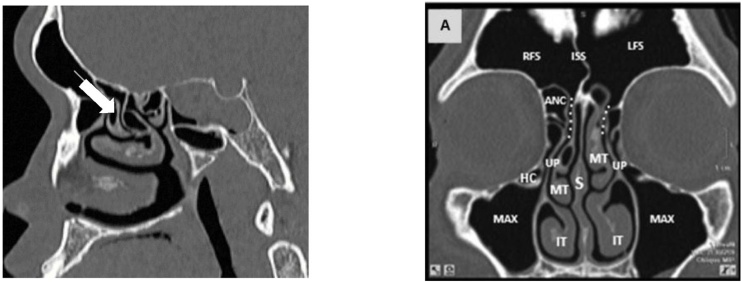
Normal anatomy of frontal recess: sagittal and coronal CT. Normal frontal recess anatomy. Sagittal CT shows the right frontal recess (white arrow). Computed tomography coronal section depicting the anatomy of the frontal sinus drainage pathway and surrounding structures. ANC, Agger Nasi Cell; RFS, Right Frontal Sinus; White dotted line frontal sinus drainage pathway; ISS, Intersinus Septum; IT, Inferior Turbinate; LFS, Left Frontal Sinus; Max, Maxillary sinus; MT, Middle Turbinate; S, Nasal Septum; HC, Haller Cell; UP, Uncinate Process.
